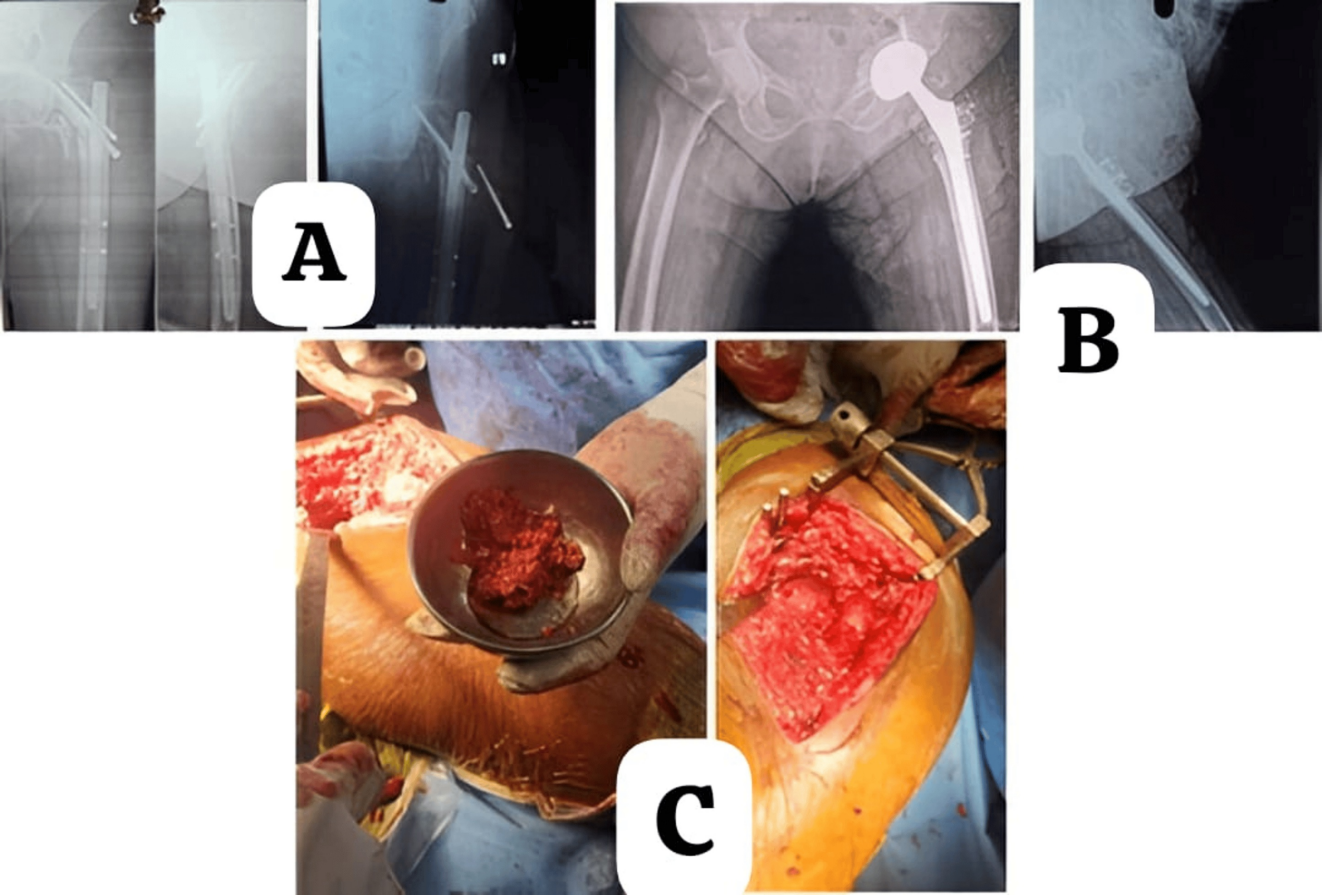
Case of a 69-year-old female with a left intertrochanteric femur fracture and failed osteosynthesis performed at another hospital. Managed with implant and femoral head removal, followed by total hip arthroplasty via the Modified Hardinge approach using autologous bone grafting in the acetabulum. (A) Preoperative X-ray demonstrating screw back-out. (B) Postoperative X-ray following total hip replacement. (C) Impaction grafting of the acetabulum using an autologous graft prepared from the femoral head with a bone mill and impacted into the acetabular floor to address a medial wall defect.
